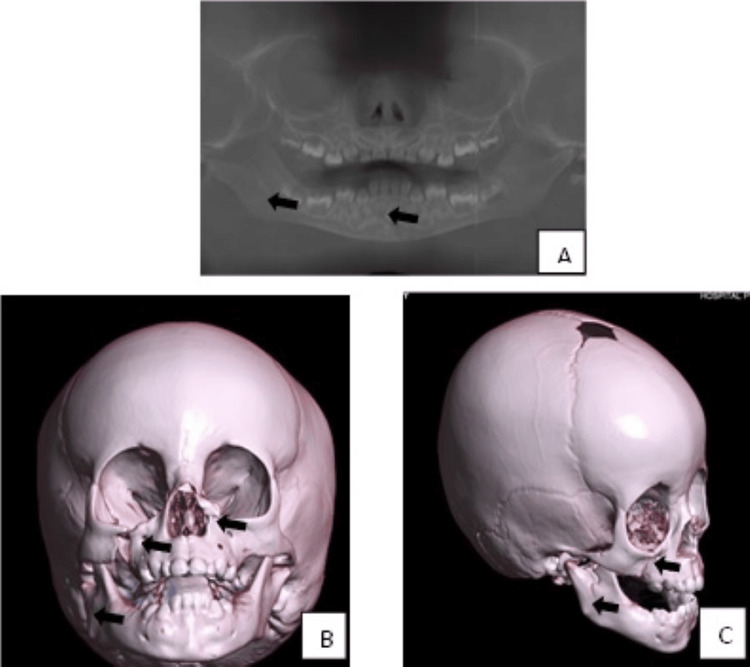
Presurgical orthopantomography and tomographic 3D reconstruction A: Orthopantomography; unicortical symphyseal fracture and high ramus mandibular condylar fracture. B and C: Simple 3D CT reconstruction; condylar fracture Kohler V, orbitomalar right Knight North V fracture, right orbital fracture, unicortical symphyseal fracture, and nasal fracture CT: computed tomography
